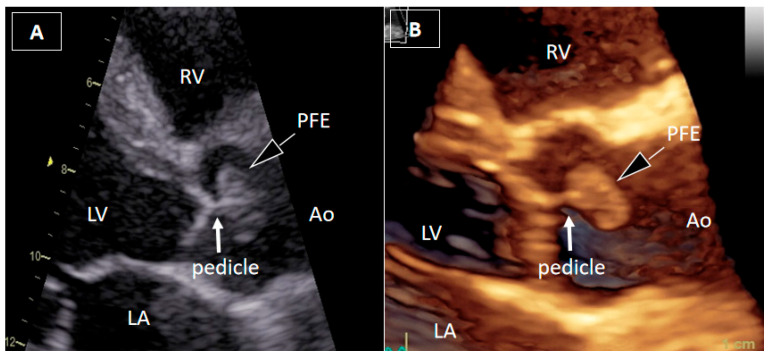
Transthoracic echocardiography. A 54-year-old man with recurrent, brief episodes of chest discomfort and no cardiovascular risk factors was referred for echocardiographic evaluation. Two-dimensional transthoracic echocardiography (2DTTE) (A) and three-dimensional transthoracic echocardiography (3DTTE) (B) demonstrated a round, mobile mass in the ascending aorta just above the aortic valve, most likely attached to the downstream surface of the right valve leaflet by a short pedicle. The attachment site of the pedicle was not clearly identified. Other structural and functional echocardiographic parameters, including myocardial strain imaging, were within normal ranges.
